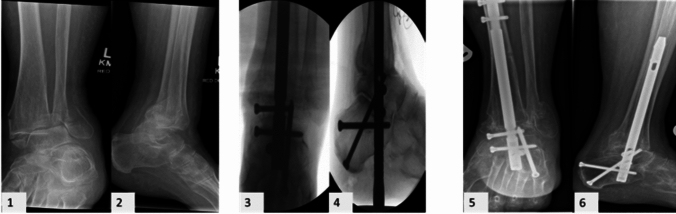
Closed isolated left comminuted ankle fracture in a female patient 87 years old sustained after a fall from standing height. (1, 2) preoperative AP and lateral radiographs of the ankle; (3, 4) intraoperative AP and lateral radiographs of the ankle demonstrating stabilization of the ankle and subtalar joint with the Phoenix Ankle Arthrodesis Nail; (5, 6) AP and lateral ankle radiographs showing ankle and subtalar fusion 4 months postoperatively
